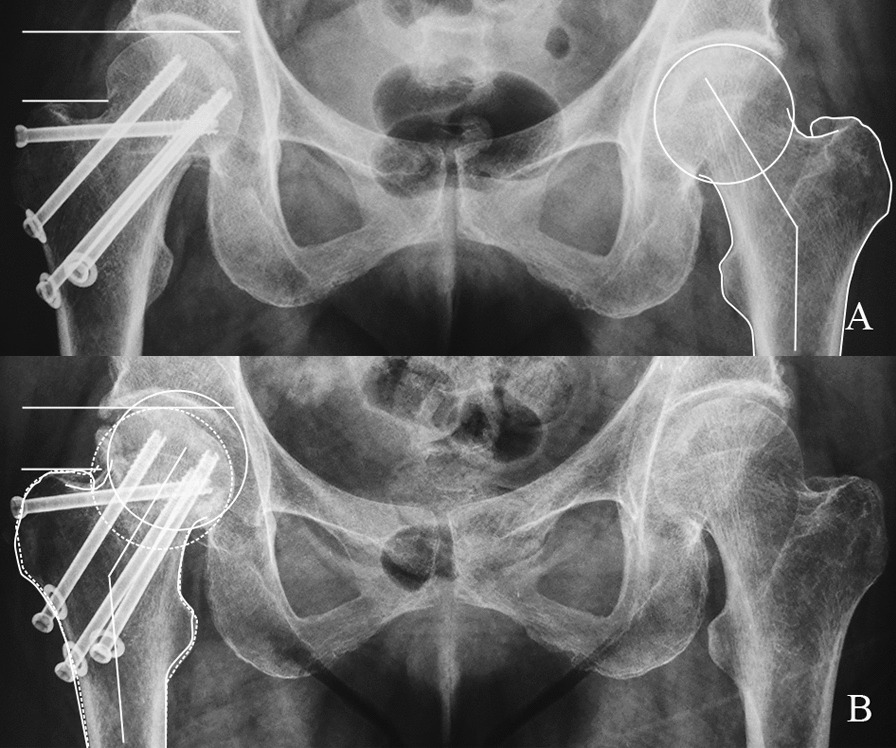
OPTCS fixation for FNF in a 47-year-old female complicated by femoral neck shortening. A AP pelvis radiograph demonstrating the uninjured proximal femur was outlined with the neck-shaft angle labeled. B 6-month postoperative radiograph demonstrates fracture union with femoral neck shortening and varus displacement. The outline of the uninjured (solid line) overlapped on the fracture side (dotted line) is provided for comparison. The screw lateral withdrawal could be detected
